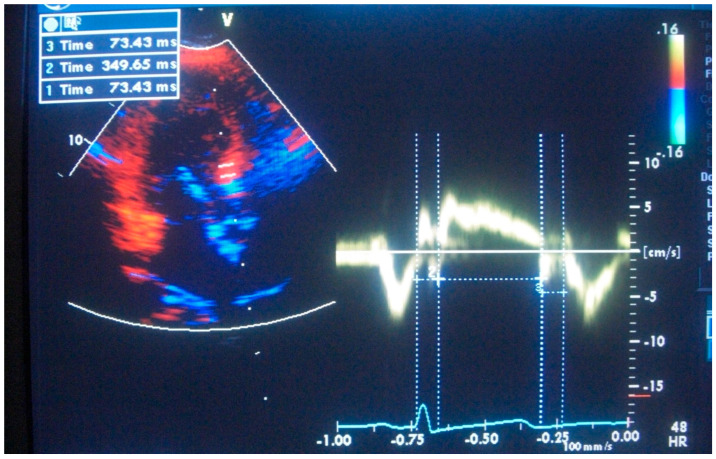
Quantitative segmental LV functional analysis of a hypertensive patient with basal septal hypertrophy using tissue Doppler echocardiography. Reprinted from ref [30].
